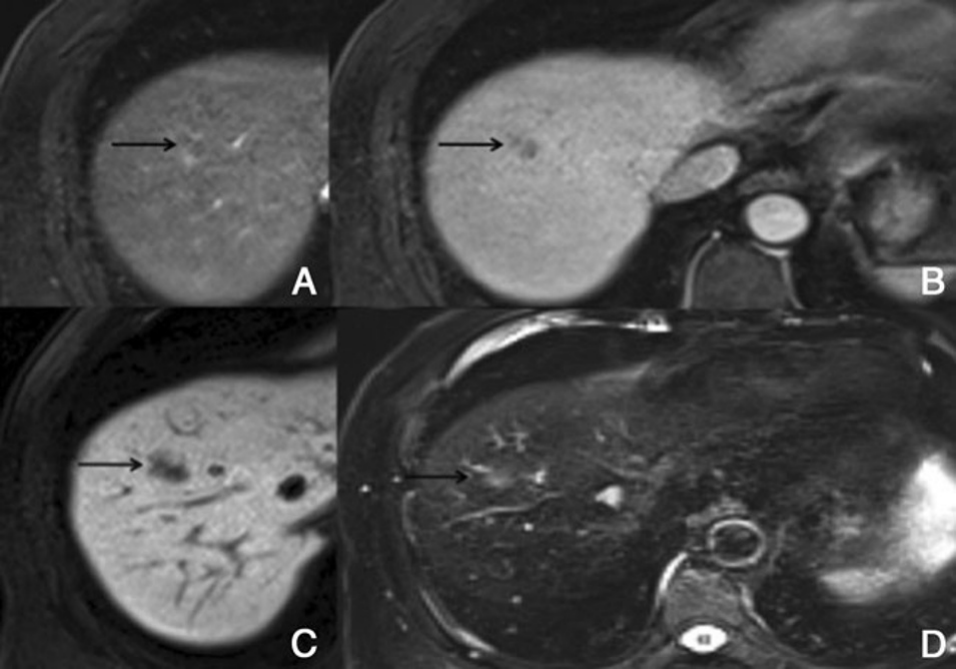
Focal hypereosinophilic necrosis nodule in a 55-year-old man with no liver disease. a Arterial phase, b PV phase, c HCP, d T2-weighted. Small non-spherical lesion with ill-defined margins in the right lobe with poor enhancement in the arterial and PV phase, mixed hypointensity on the HCP and slightly hyperintense in T2-weighted (*black arrows*). This lesion was biopsy proven to be a lesion of focal eosinophilic infiltration
